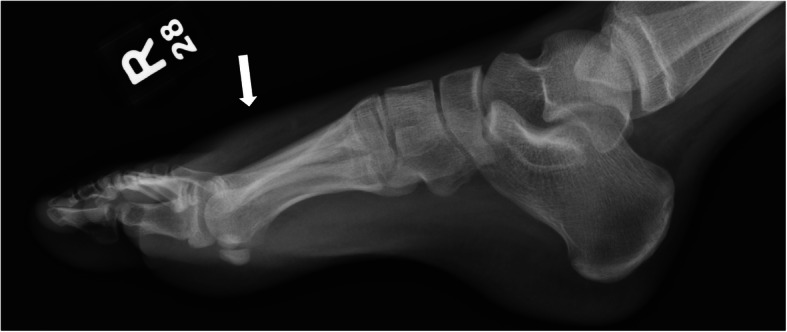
Right foot radiograph demonstrated mild soft tissue swelling and fat stranding (white arrow) which can be seen with cellulitis or edema. No definite radiographic evidence of osteomyelitis was identified
